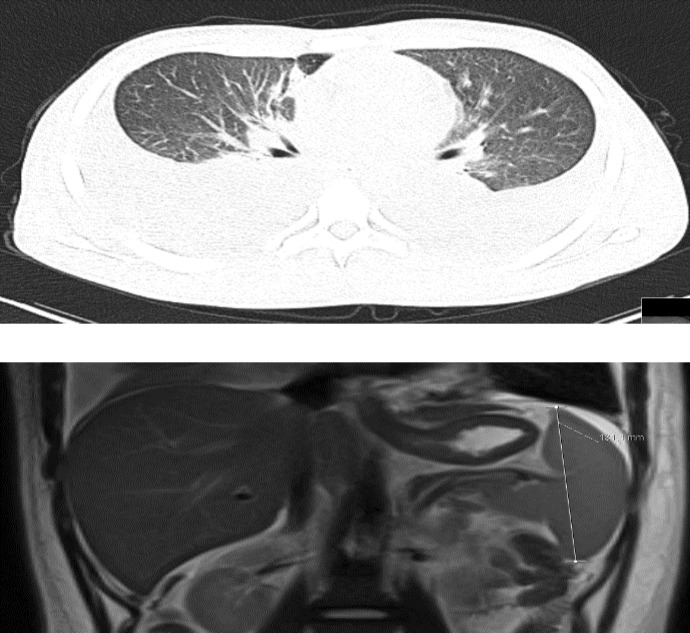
Bilateral parenchymal ground-glass opacities, patchy rounded areas of consolidation, and interlobular septal thickening were detected on CT scan. A mild splenomegaly was shown on MRI scan.
